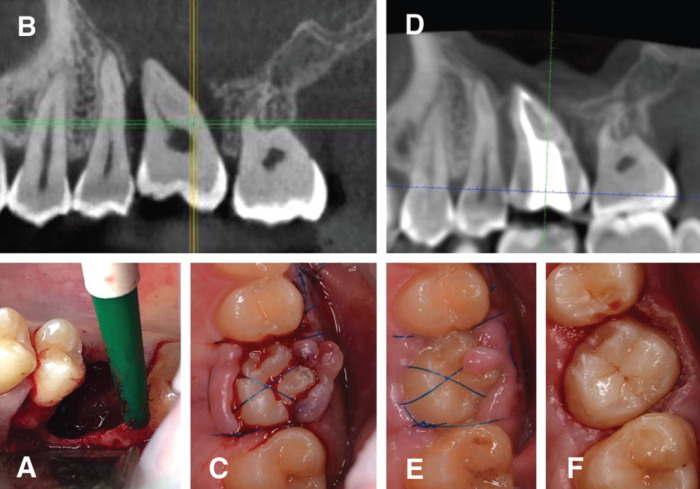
A. Revealing of a local vertical bone tissue loss. B. CBCT scans after surgery. C. Situation after suturing. D. CBCT scans after endodontic treatment. E. Condition of soft tissue, 14 days after surgery. F. Soft tissue excision made by diathermic coagulator.
